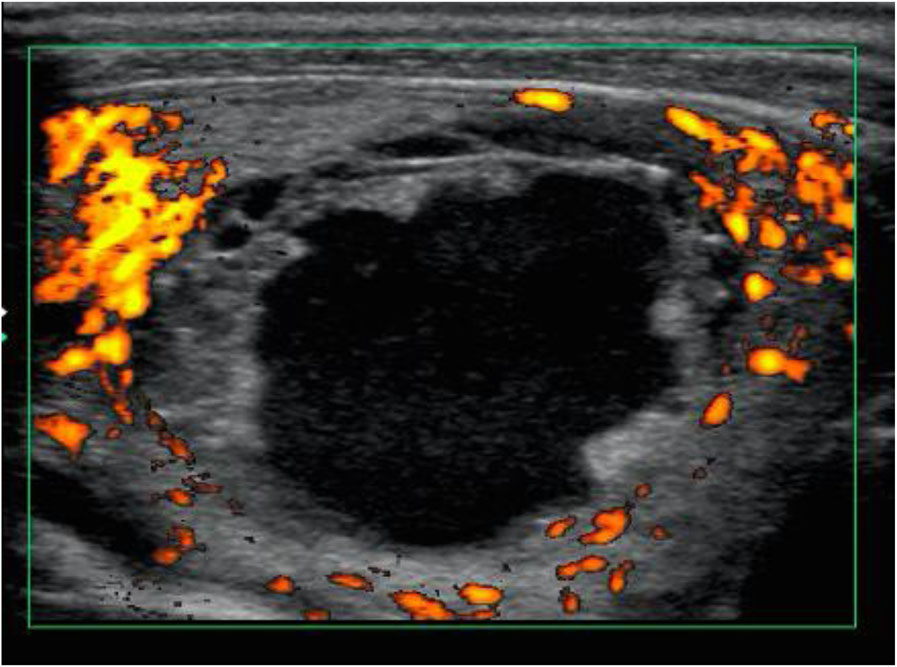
Ultrasound examination of the thyroid gland on admission day 4 showing a thyroid nodule in the right lobe surrounded by enriched thyroid vessels
